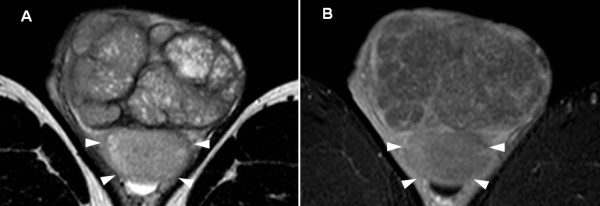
Magnetic resonance imaging of the left testis. (A) T2-weighed image showing a large multinodular tumor with heterogeneous high intensity. (B) Dynamic contrast-enhanced subtraction showing that the left testis had only slight enhancement. There seemed to be a normal left testis (arrowheads), although the border between the tumor and normal testis was not very clear.
